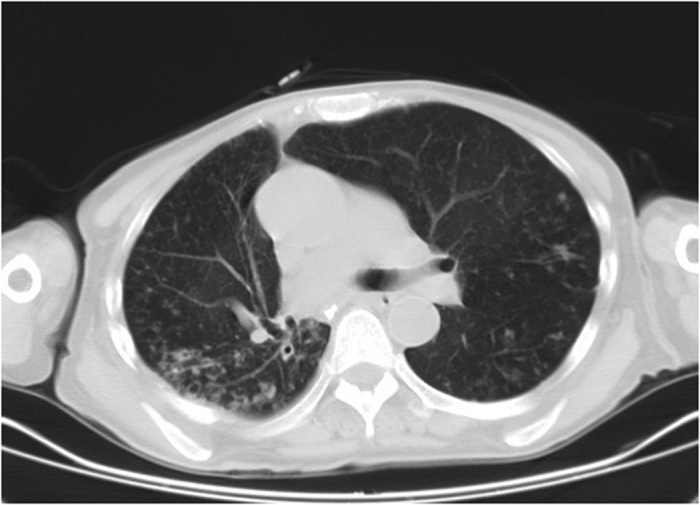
M. abscessus* lung disease in a 62-year-old woman. Chest CT scan shows centrilobular nodules and bronchiectasis. Also note lesions without segment or lobe predominance.
